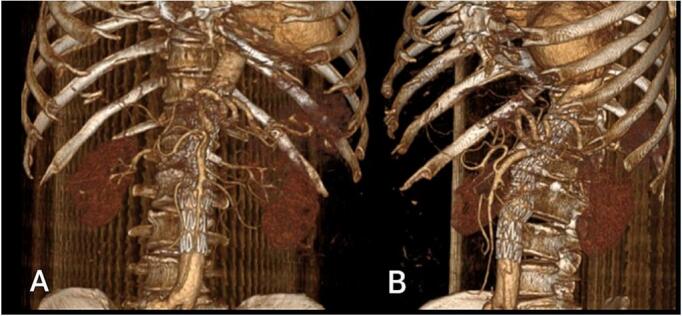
Post-procedural CTA after three months demonstrating the successful exclusion of the pseudoaneurysm with four-fenestrated EVAR performed on case 1. Each target vessel remains patent, with no observed endoleak. A) Anterior view B) lateral view.
